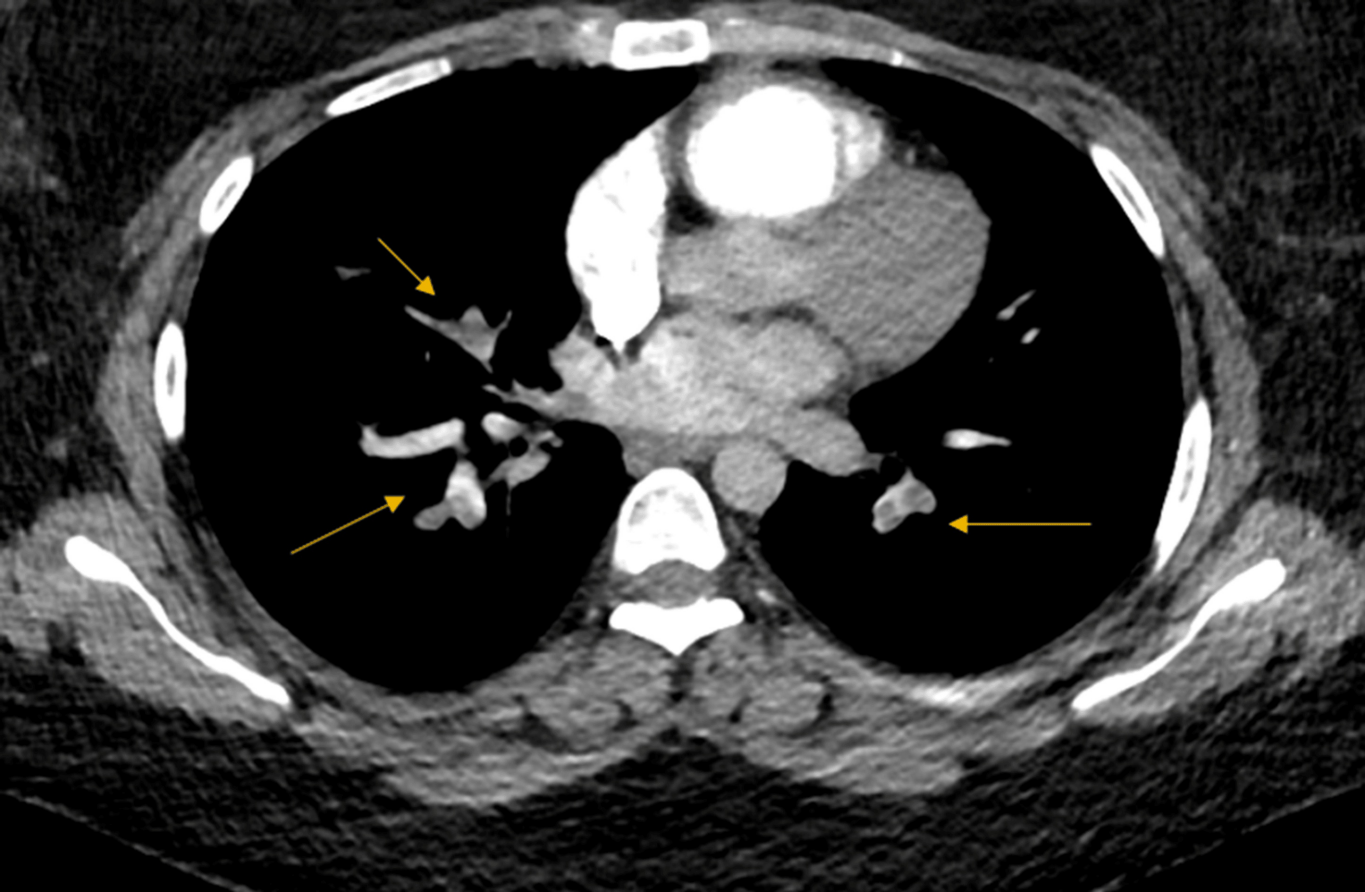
CTPA showing multiple bilateral lobar and segmental pulmonary emboli (yellow arrows) CTPA: computed tomography pulmonary angiography
